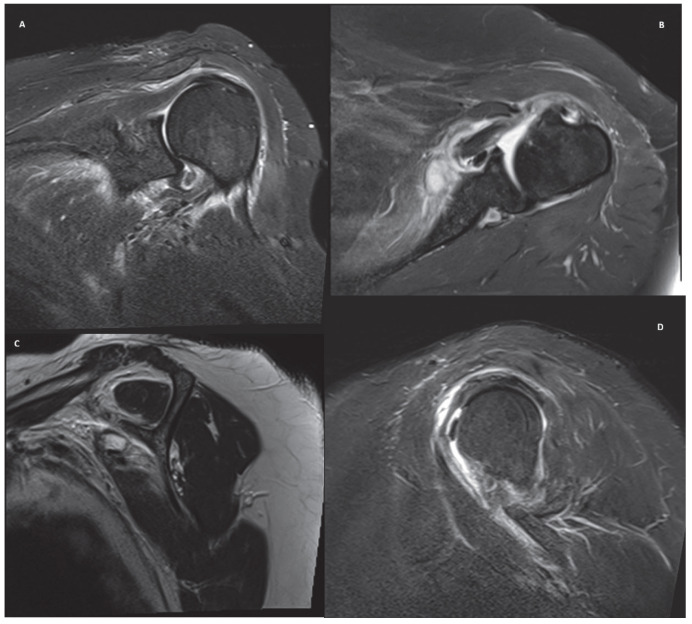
MRI images of an isolated Lafosse type 2 subscapularis tear in coronal (A), axial (B) and sagittal (C,D) view.
